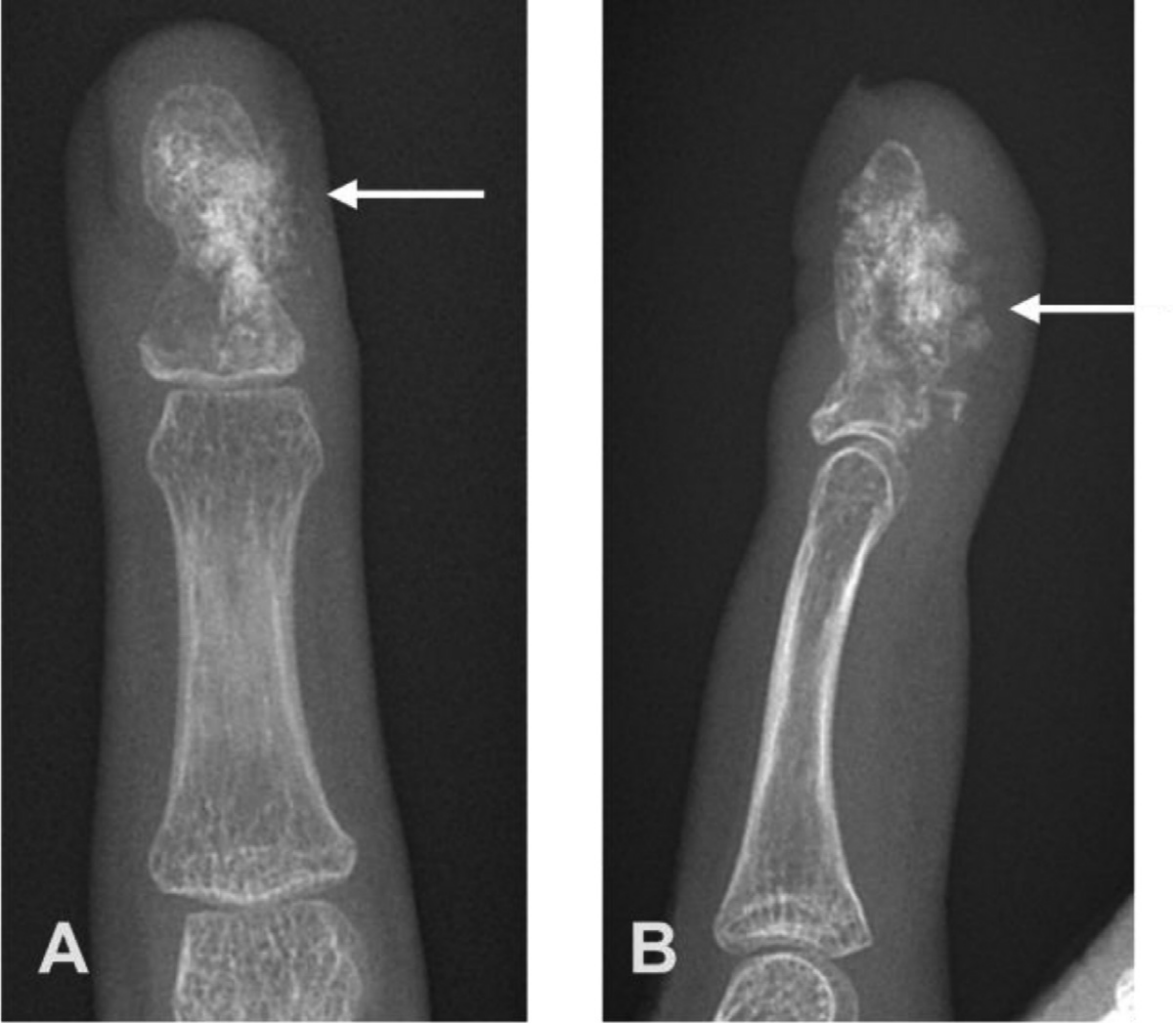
(A) PA and (B) lateral radiographs of the left hand fourth distal phalanx 10 weeks following biopsy and curettage, demonstrating postsurgical changes from open curettage and grafting (arrow).
